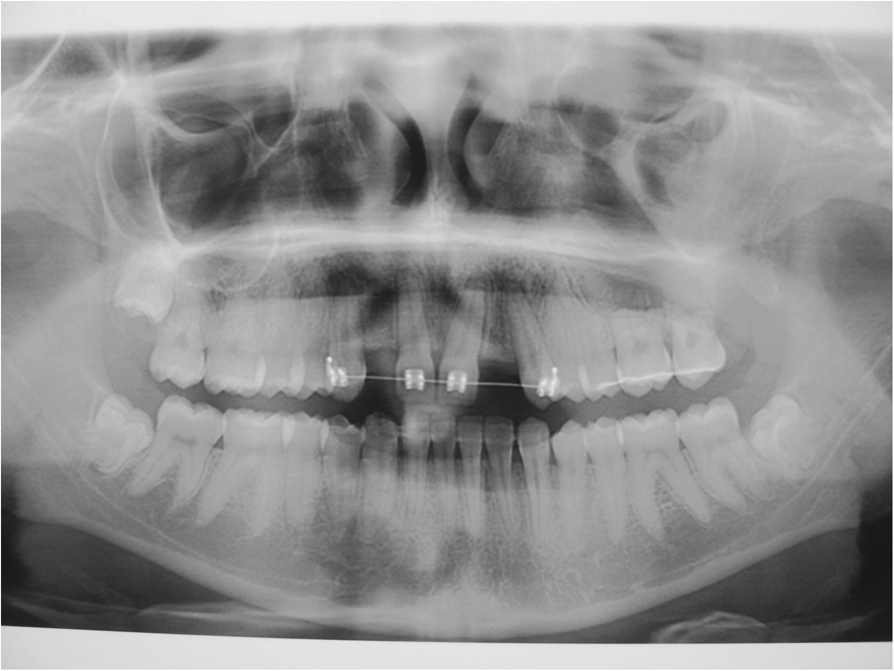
Orthopantomography follow-up after 6 months.
